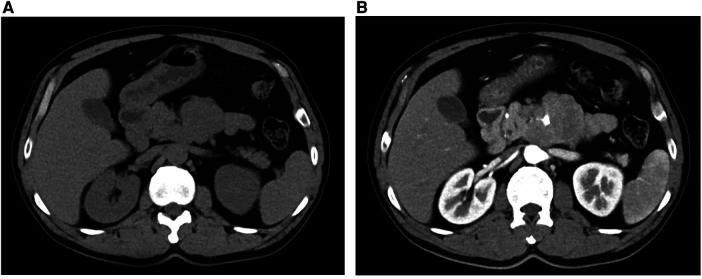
CT (A) and contrast-enhanced CT (B) showing an occupying lesion below the neck of the pancreas.
